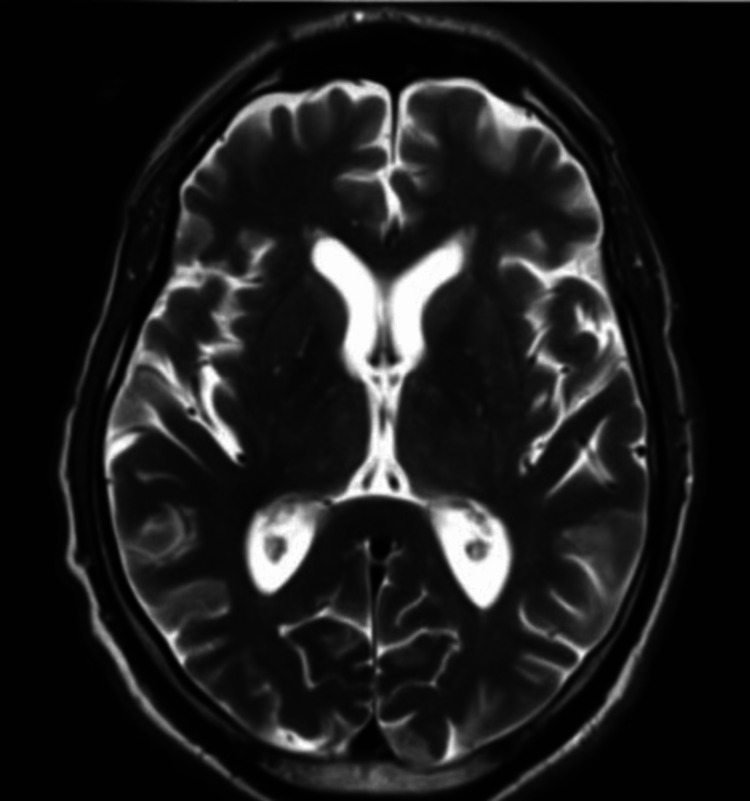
MRI brain: Day-14 axial T2-weighted image shows complete resolution of lesions noted on day one
